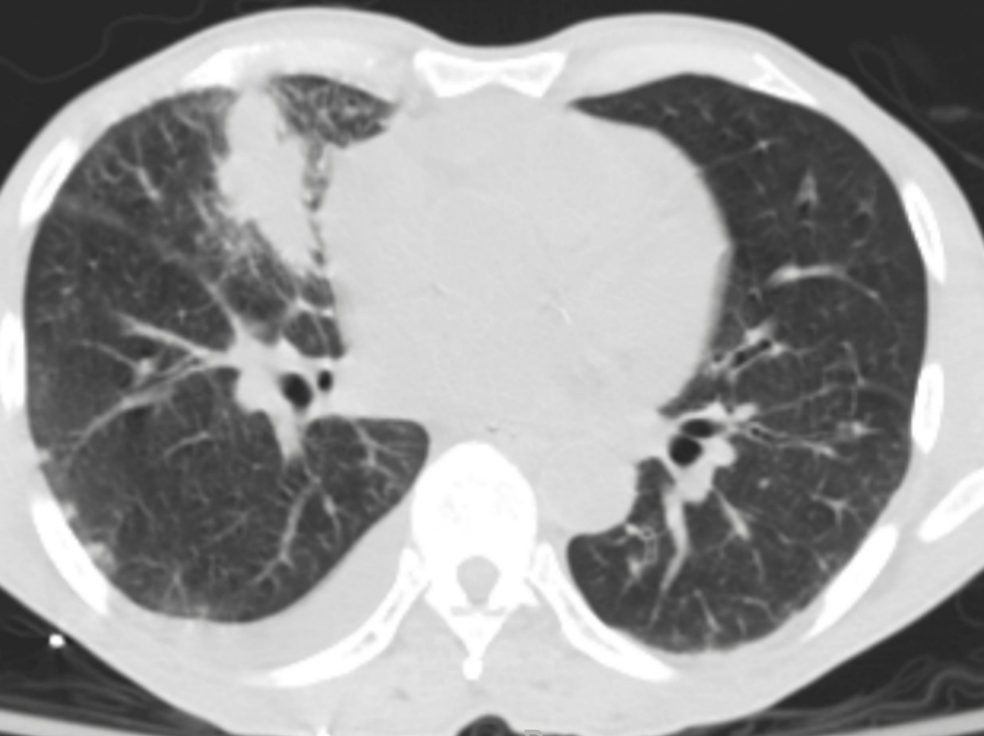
Chest computed tomography of the patient. CT scan of the whole chest showed a mass-like opacification in the right middle lobe, diffuse interlobular septal thickening throughout the right lung, and several nodular foci in the right lung.
